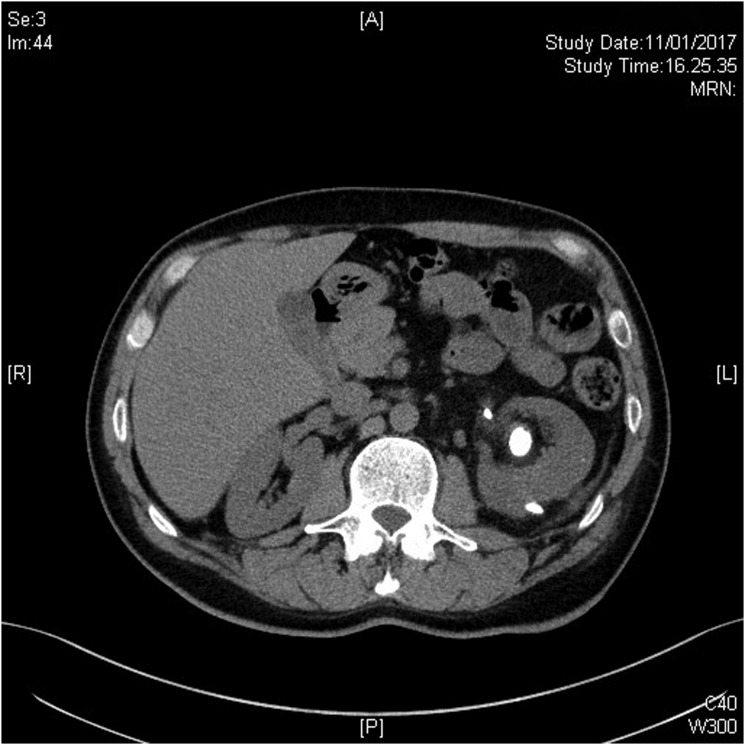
CT scan showing subcapsular kidney collection healing after 7 days of drainage.
